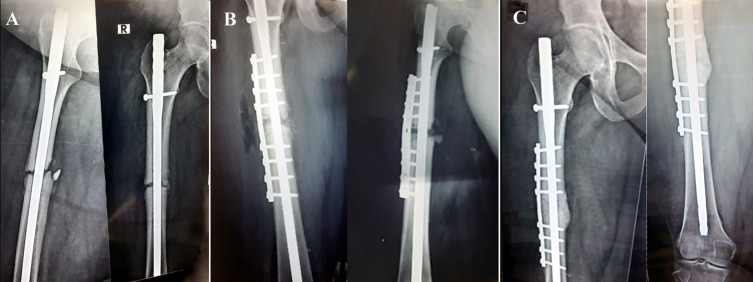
Twenty-three year-old lady with atrophic nonunion fracture shaft Rt femur fixed by intramedullary interlocking nail. A. Preoperative X-ray, 9 months after nail fixation, showing; atrophic nonunion at the fracture site. B. Immediate postoperative X-ray after plate and graft augmentation. The fracture was fixed by broad DCP and 8 bicortical screws. The graft can be seen filling the nonunion site. C. 3 months postoperative X-ray showing; complete bone union with good consolidation at fracture site.
